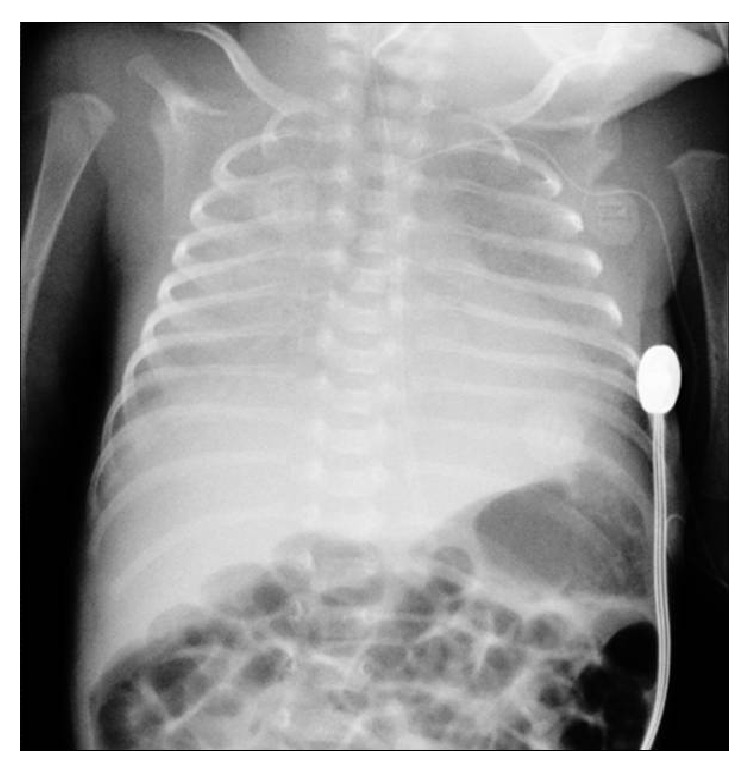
Chest radiograph (20 days of life) showing bilateral diffuse alveolar opacities.
